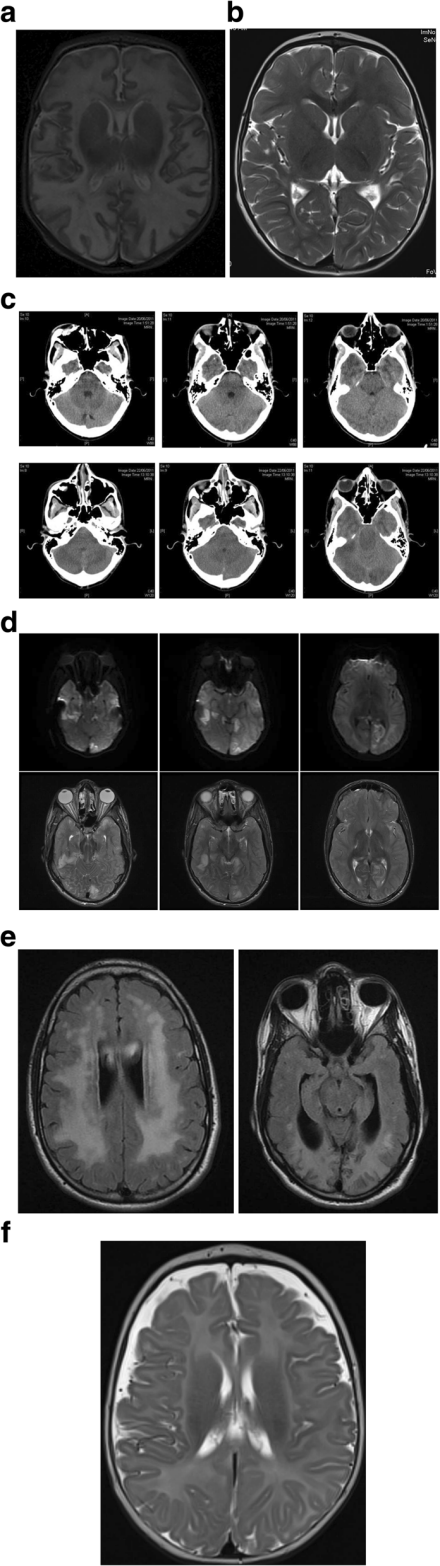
a) Sagittal MRI Image of the brain on day 12 of case I with CACTD, indicating extensive abnormal T2 hyperintensity seen within the white matter of both cerebral hemispheres. b) T2 -weighted MRI of brain of case 1 at 16 months indicating mild white matter and cortical volume loss especially in the parietal region. Hyperintensity has substantially improved
